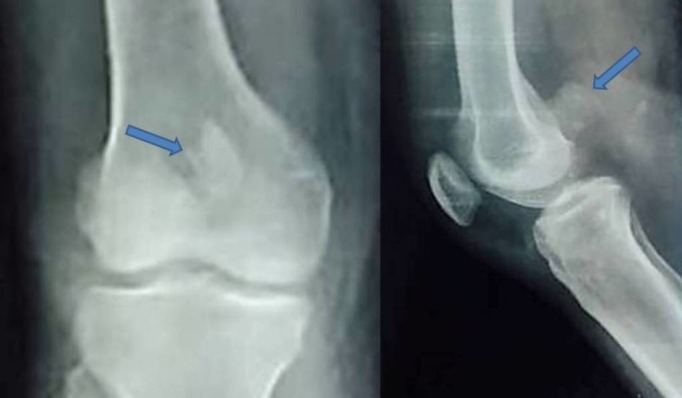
The pre-op Xray images of the anteroposterior & lateral views showing the distal femur medial condyle Hoffa type II(C) fracture
